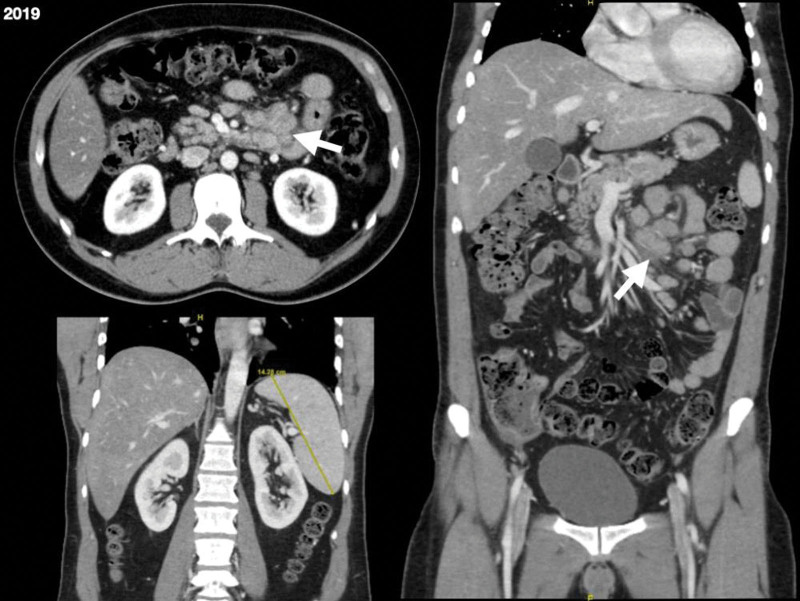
Images of the CT-scan performed in February 2019. White arrows show mesenteric lymph node enlargement. Mild splenomegaly (14 cm) is also indicated.
